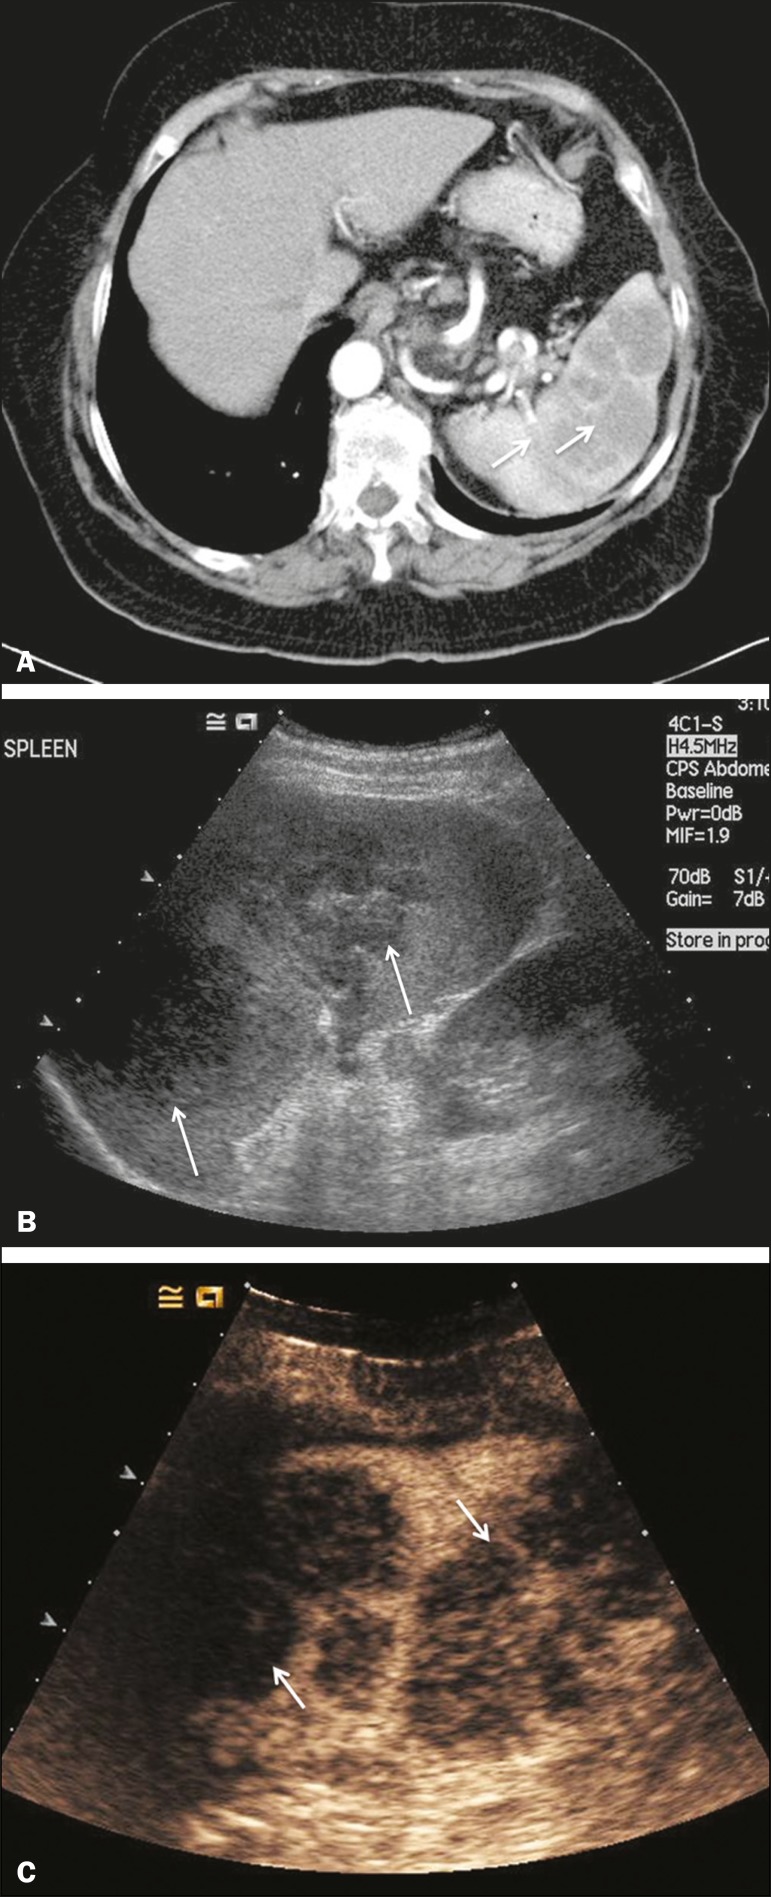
Multiple splenic lymphoma. A: Contrast-enhanced CT scan
